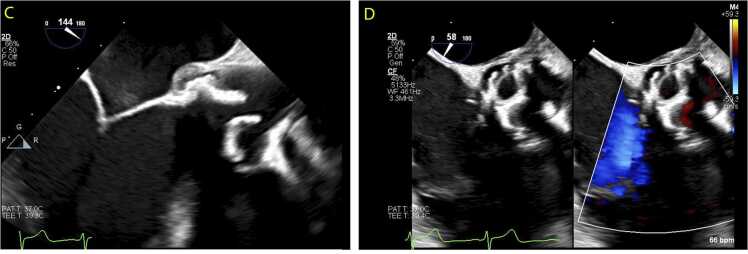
Transesophageal echocardiogram long axis (C) and short axis (D) views showing resolution of previously noted vegetation after 6 weeks of antibiotics.
